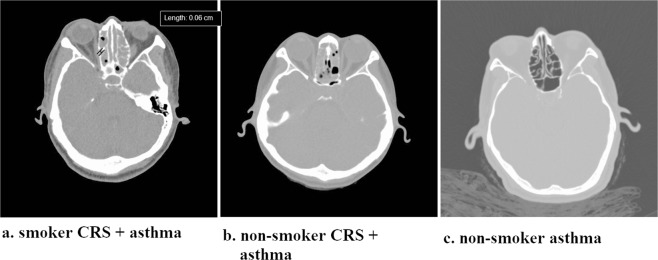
Non-contrast sinus computed tomography (CT). The maximal thickness of the bony partition of the anterior ethmoid sinuses (indicated by caliper) was measured on an axial sinus CT section. caliper
